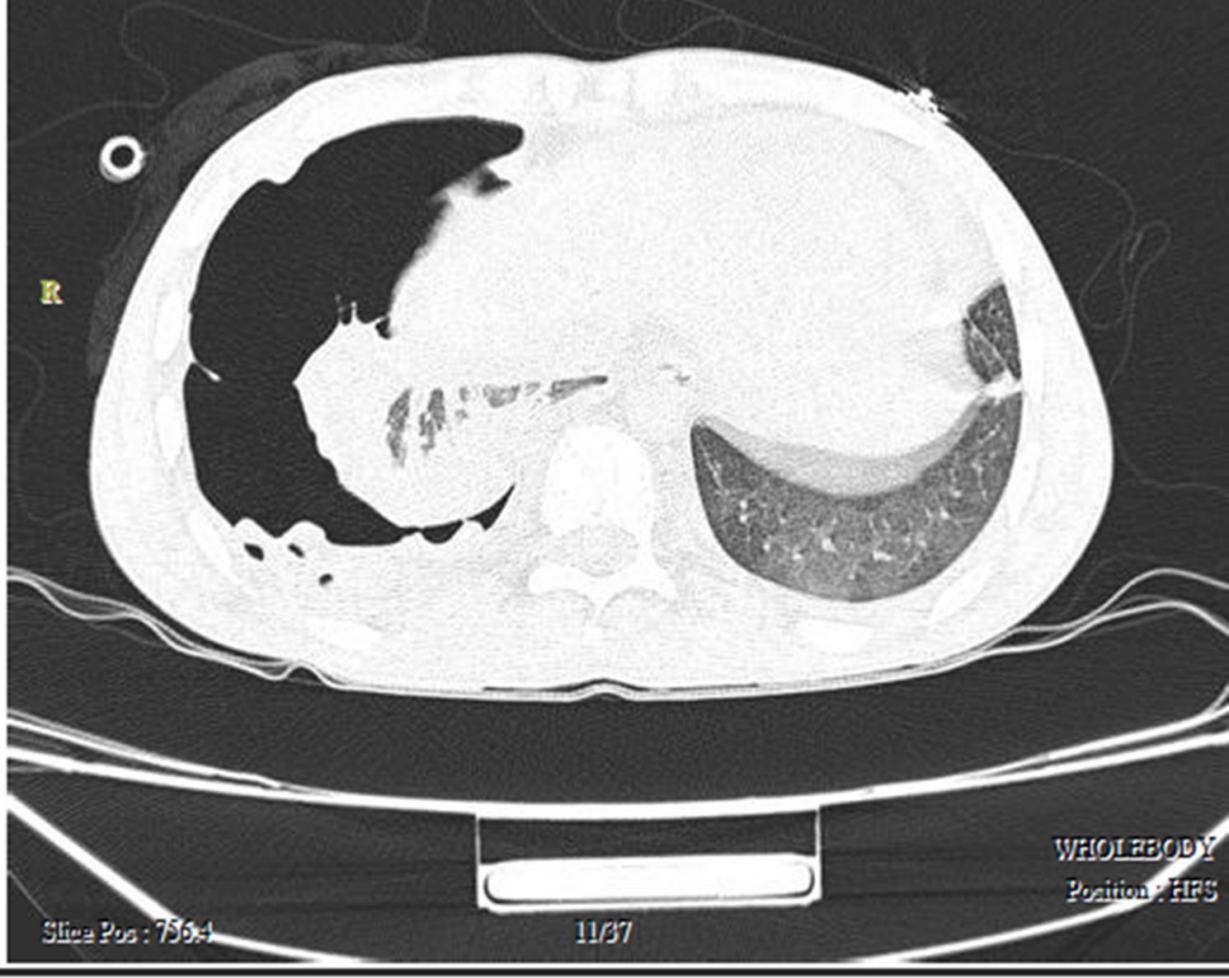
Chest CT scan of Case 1 demonstrating the classic water‐lily sign.
